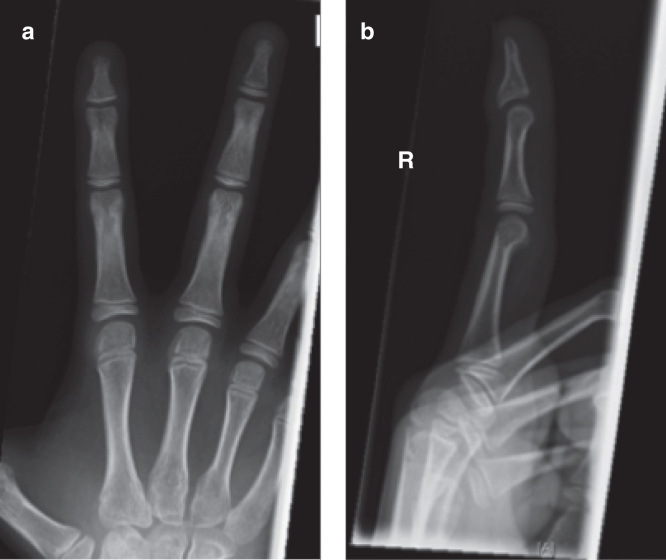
Radiographs at 2 years demonstrating good coronal alignment on the anteroposterior view (*a*). However, premature fusion of the physis has occurred with a degree of malunion on the lateral view (*b*).
